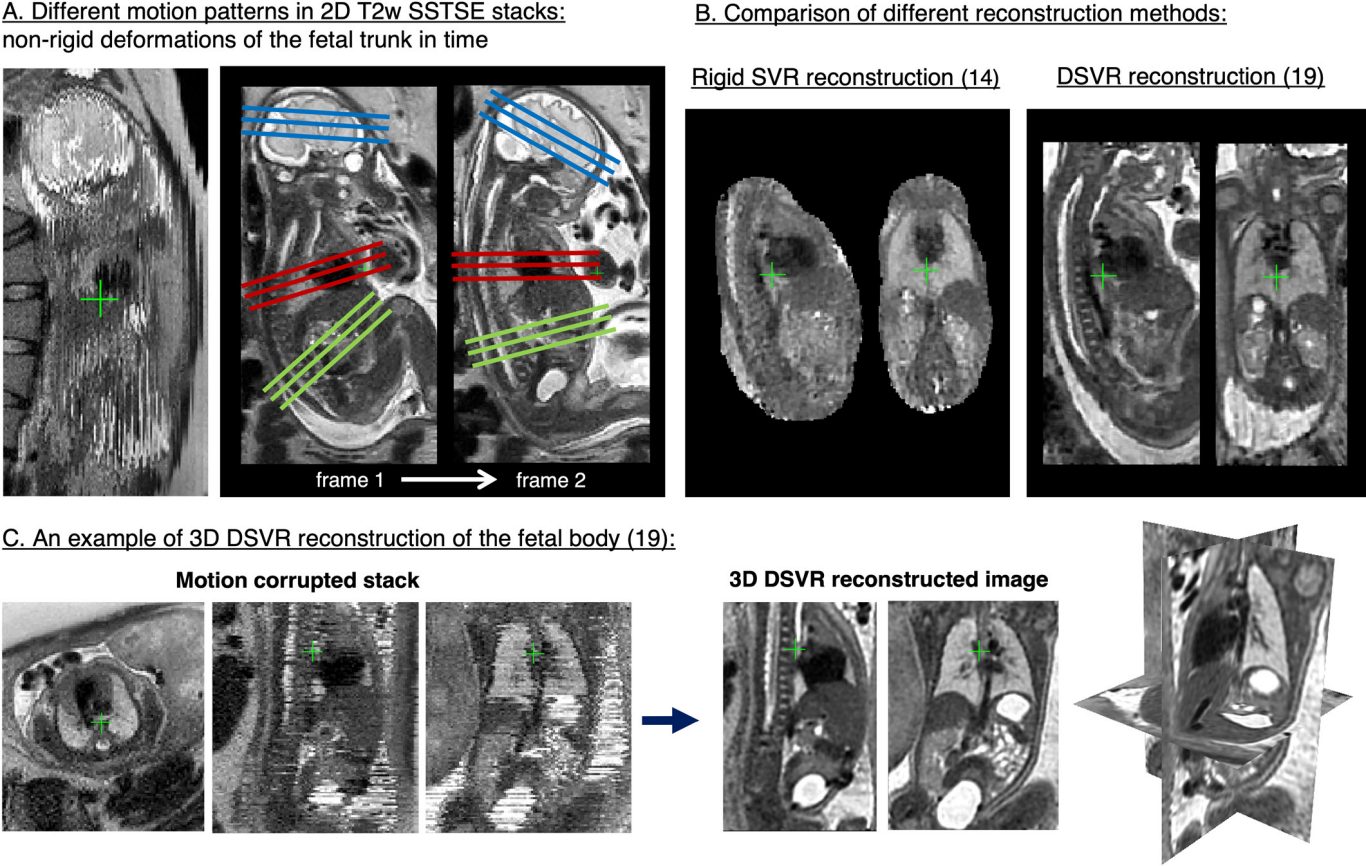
3D DSVR reconstruction for foetal body MRI. These examples are based on the MRI datasets acquired at St.Thomas’ Hospital, London. DSVR, deformable SVR; SVR, slice-to-volume registration.
